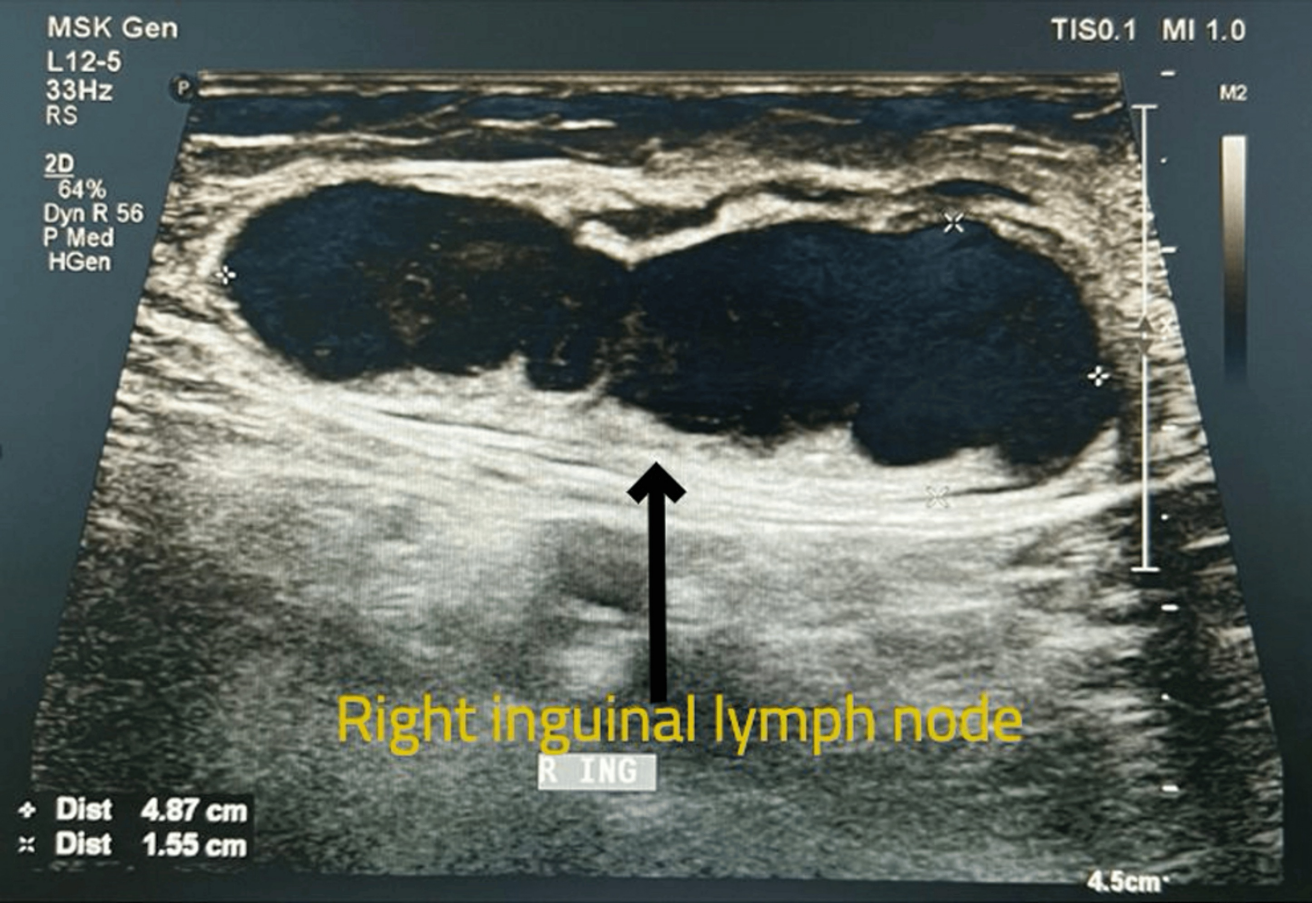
USG showing enlarged lymph nodes in the right inguinal region USG: ultrasonogram The black arrow shows an enlarged right inguinal lymph node.
